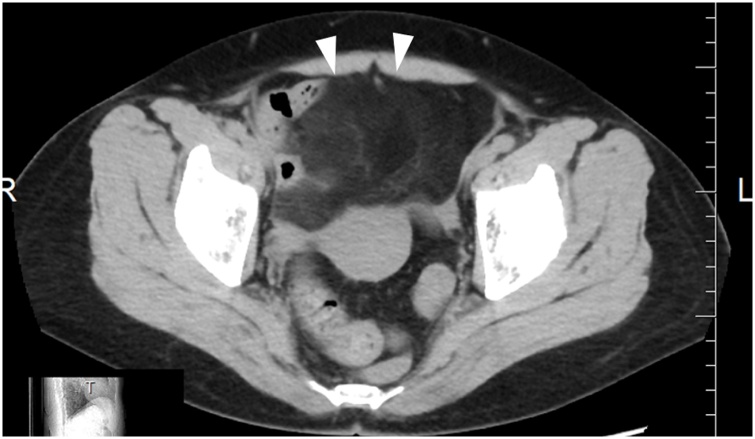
Abdominal CT showed an 8 × 8 × 4 cm mass (arrowhead) on the cranial side of the bladder in the abdomen.
